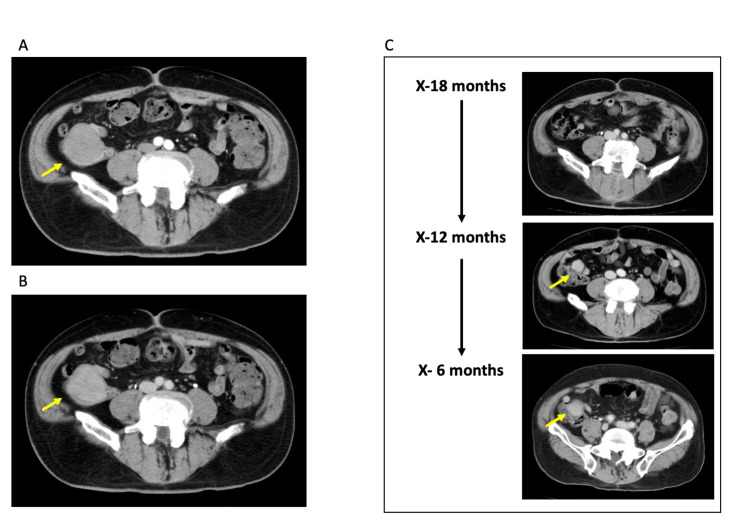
Contrast-enhanced CT (CECT) A, B: Contrast-enhanced CT (CECT) (A: early phase, B: late phase). CECT shows a dense tumor in the ileocecal area. The tumor represents mild vascularity. C: Chronological alteration of the ileocecal tumor. The time point X stands for the date when the ileocecal tumor was noticed. Retrospectively the tumor was evident at the time point X-12 months. The tumor showed an expansive growth retrospectively.
